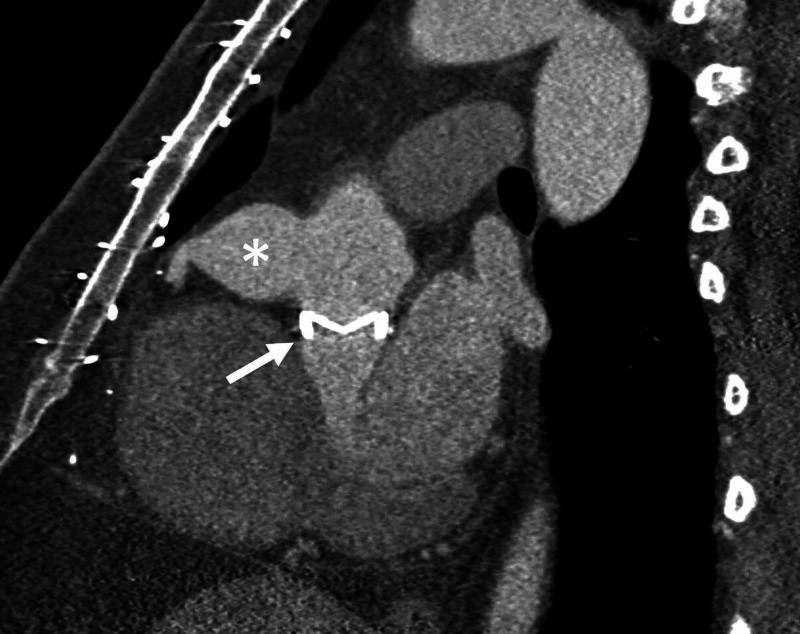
Multiplanar Reconstruction Sagittal CTA Image Prosthetic aortic valve replacement (Carbomedics 27 mm valve) (white arrow) and the aneurysm of the proximal right coronary artery and ostial dilatation (asterisk).
